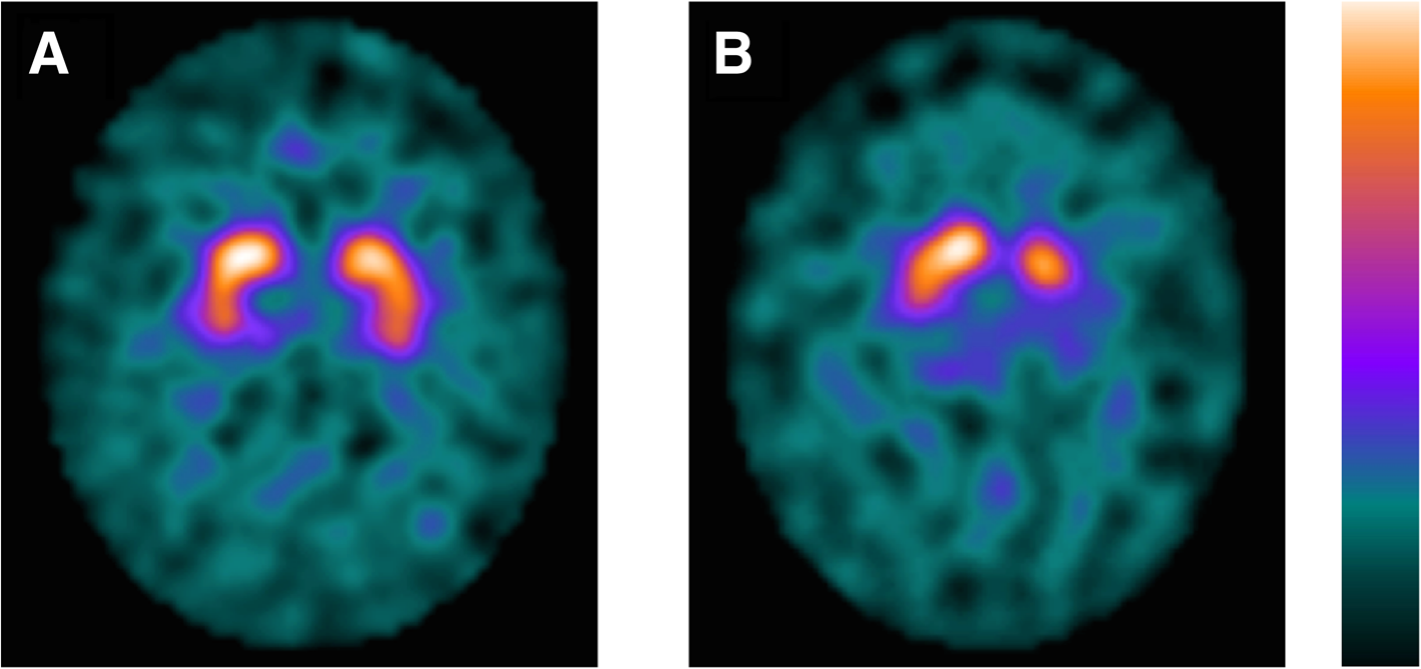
DAT SPECT imaging. Normal (a) and abnormal (b) [^123^I]FP-CIT SPECT imaging of patients in the LEAP cohort. Patient A is a 64-year-old male. Patient B is a 63-year-old female. DAT, dopamine transporter; SPECT; single-photon emission computed tomography; LEAP, Levodopa in EArly Parkinson’s disease
